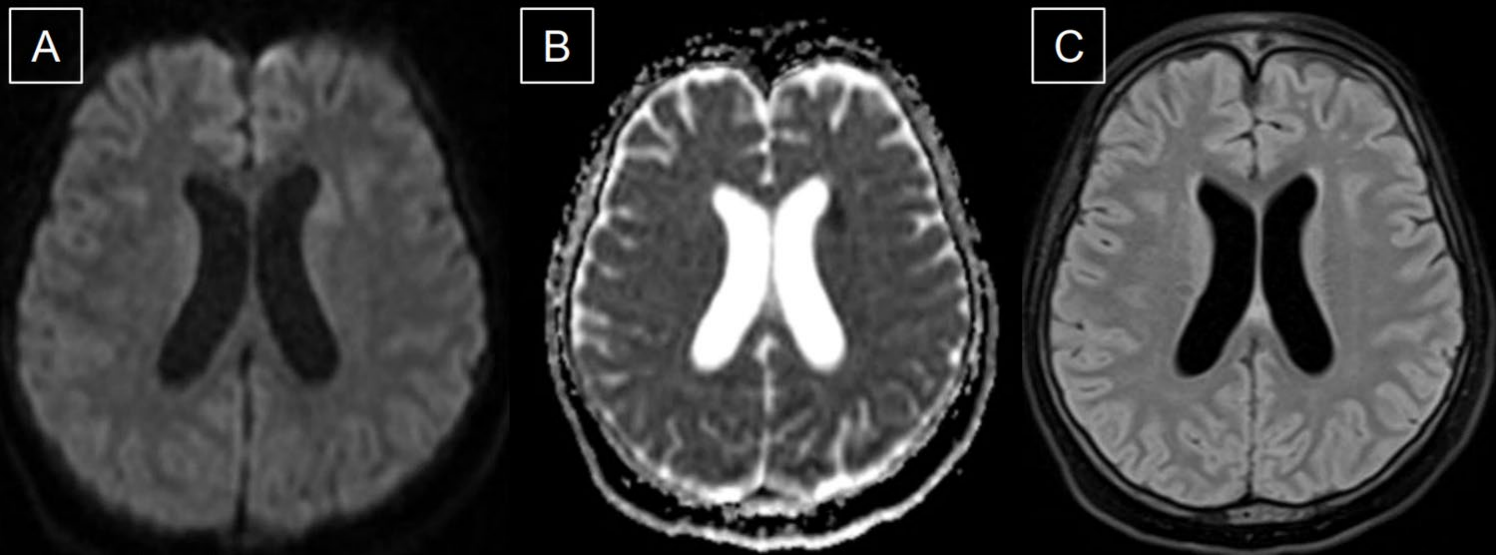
Diffusion-weighted magnetic resonance (MR) image of the brain (A), demonstrates a small area of hyperintensity in the anterior left periventricular white matter, which corresponds with hypointensity on the apparent diffusions coefficient (ADC) map (B). Of note, there is no signal change on the fluid-attenuated inversion recovery (FLAIR) image (C) in this acute infarct
